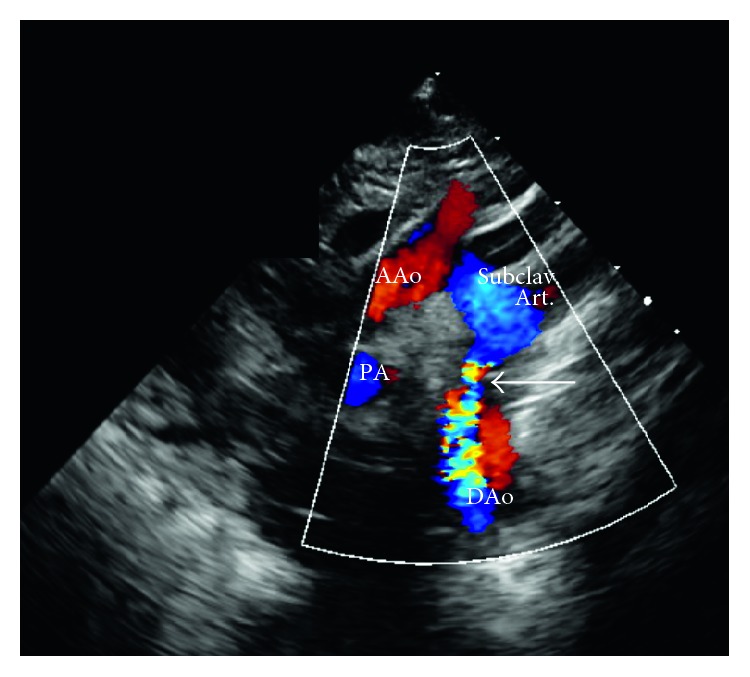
Color Doppler showing narrowing (arrow) with marked flow acceleration in descending aorta. AAo: ascending aorta; DAo: descending aorta.
